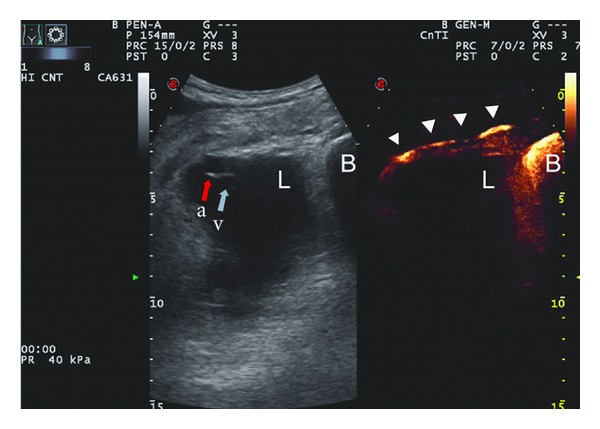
The simultaneous visualization of the same region in conventional B mode and contrast-enhanced mode allowed the identification of ureters. Left side: B mode ultrasound visualization of renal artery (a) vein (v) within the lymphocele (L) and bladder (B). Right side: contrast-enhanced ultrasound visualization of the same region showing the bladder (B) and the ureter (arrows) filled by contrast agent.
